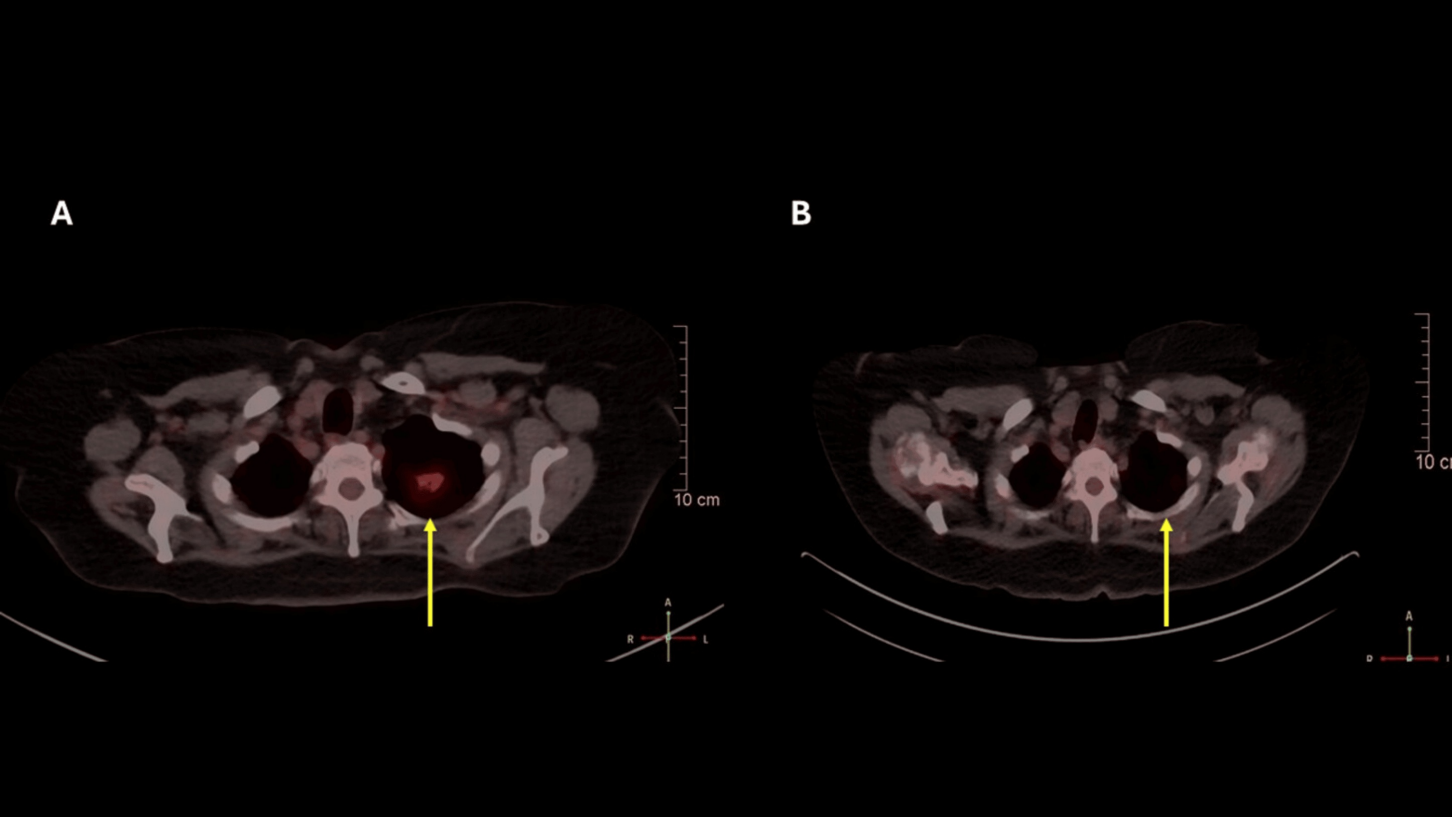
Positron Emission Tomography Before Surgery and After Pembrolizumab Therapy. A: A hypermetabolic nodular lesion is observed (maximum standardized uptake value: 4.6), with spiculated margins, pleural contact, and an internal air bronchogram, measuring 33 × 29 × 22 millimeters, located in the left posterior apical segment. B: No hypermetabolic lesions are detected on the six-month oncologic follow-up positron emission tomography-computed tomography after pembrolizumab therapy.
